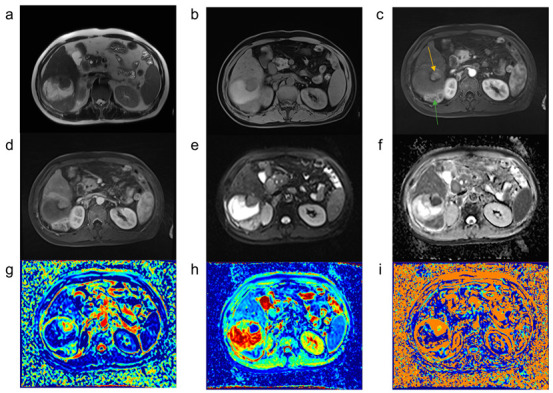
Magnetic resonance images of hepatocellular carcinoma treated using TACE. Most of the tumors after TACE treatment were necrotic, with the tumor active area (orange and green arrows) visible on the edge, which was significantly enhanced at the arterial phase, and the DWI image also showed a high signal intensity. (a) T2-weighted image; (b) T1-weighted image; (c,d) MR enhanced images; (e) diffusion-weighted image with a b value of 0 s/mm^2^; (f) ADC image; (g–i) parametric maps (*f*, D, and D*, respectively) calculated from the IVIM.
